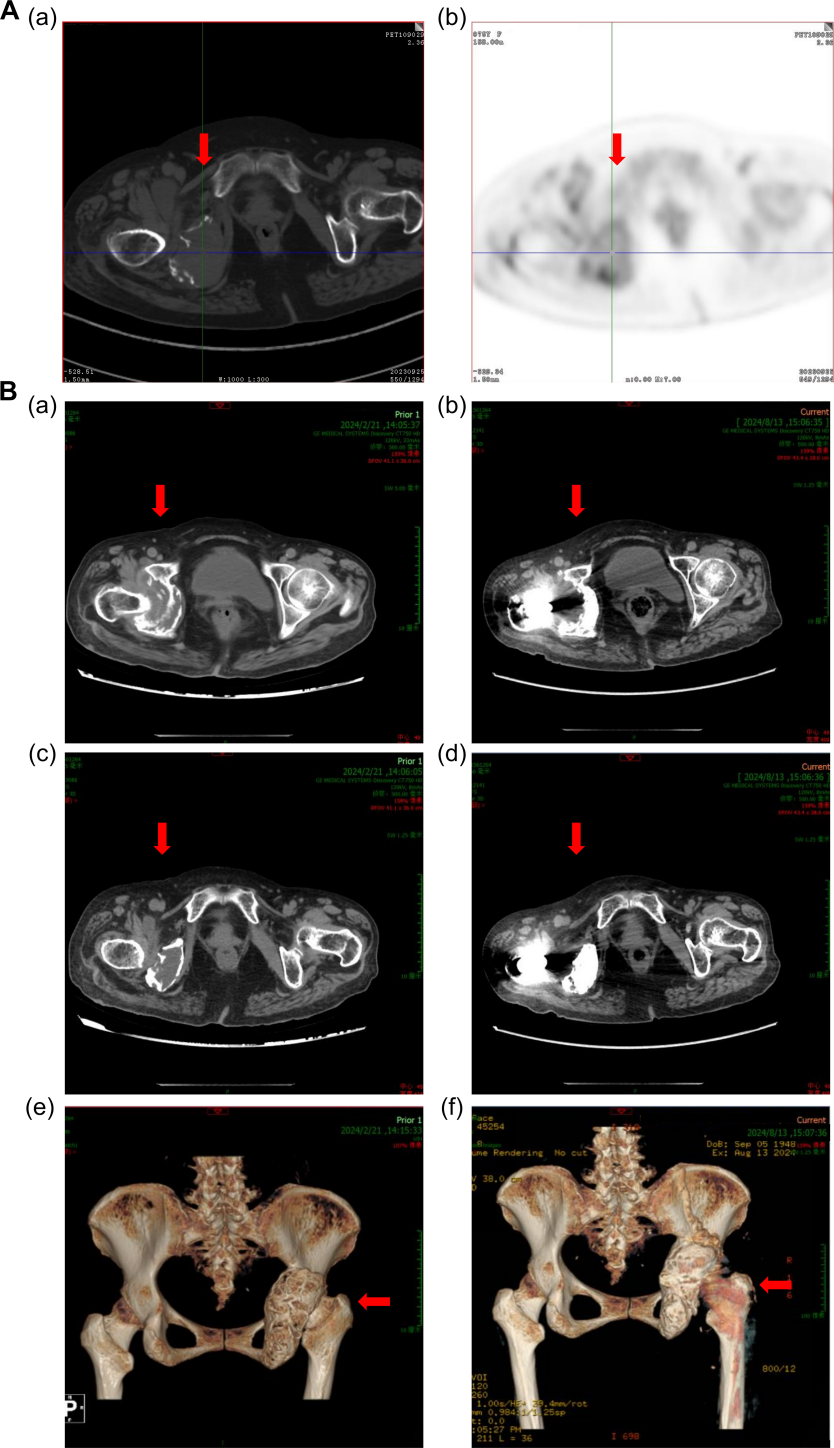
Computed tomography images of the right hip joint. (A) Computed tomography images show an extramedullary mass in the patient’s right hip joint in September 2023. (B) Reduction in the size of the extramedullary mass after 3 months of anti-BCMA CAR-T cell therapy, as shown in (a), (c), and (e) in February 2024. (c) Computed tomography images of the right hip joint after right artificial femoral head replacement, as shown in (b), (d), and (f) in August 2024. The red arrows in the figure indicate the lesions or surgical site.
